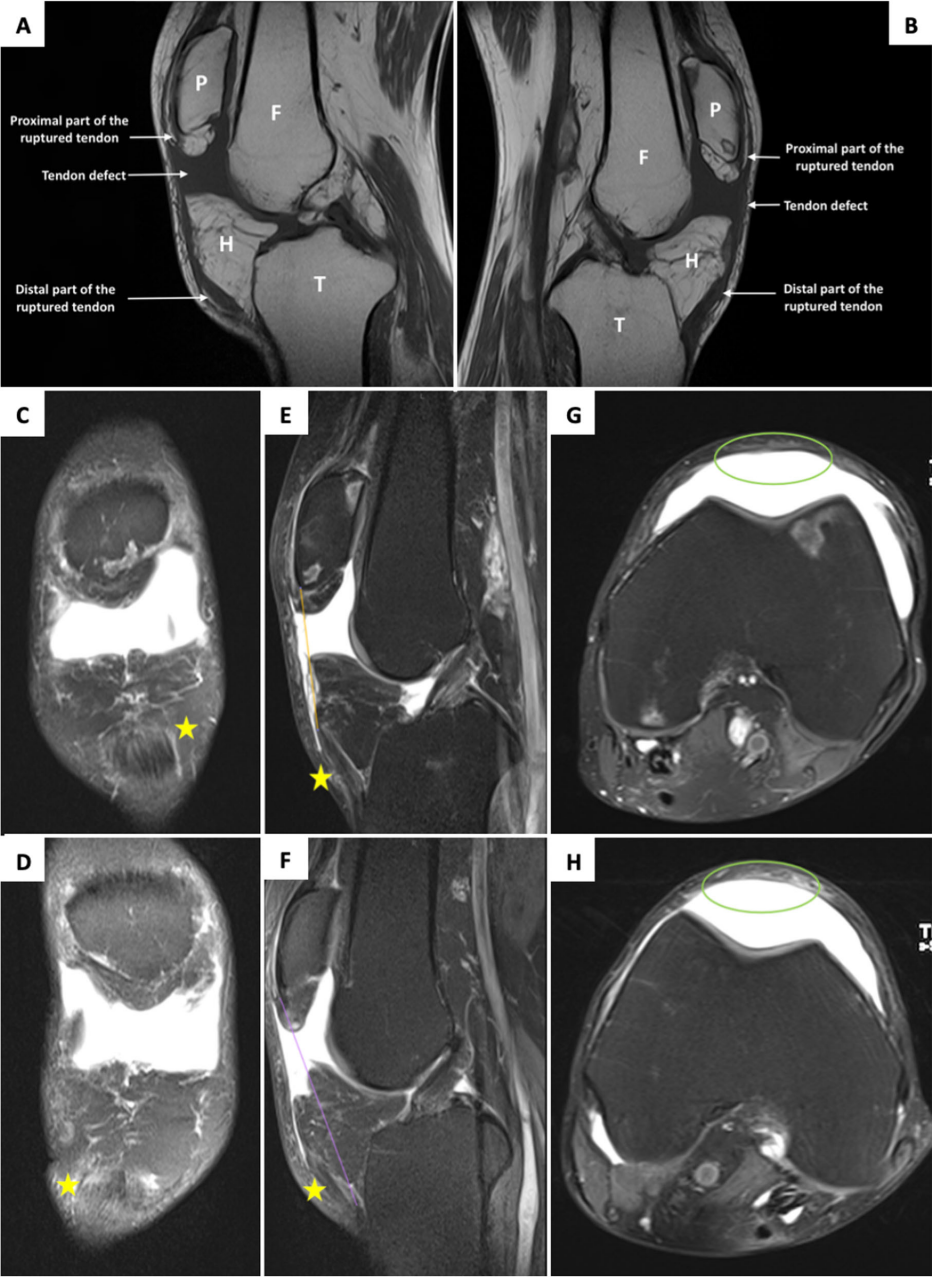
Sagittal T1-weighted MRI showing the rupture of the patellar tendon in the right (a) and left (b) knees (P: patella, F: femur, T: tibia, H: Hoffa’s fat pad). Proton Density Fat-Saturation MRI sequences (c: right coronal, d: left coronal, e: right sagittal, f: left sagittal, g: right axial, h: left axial) showing distal patellar tendons stumps (yellow stars), the diastasis and loss of substance of the patellar tendon (green ellipses)
